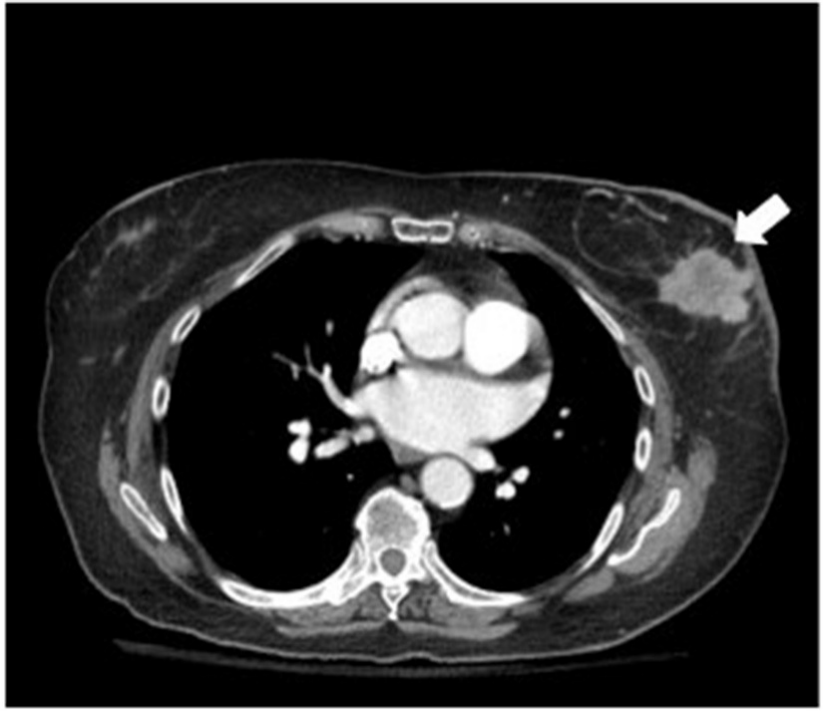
Chest CT showed a 4-cm left-breast mass with central necrosis.
